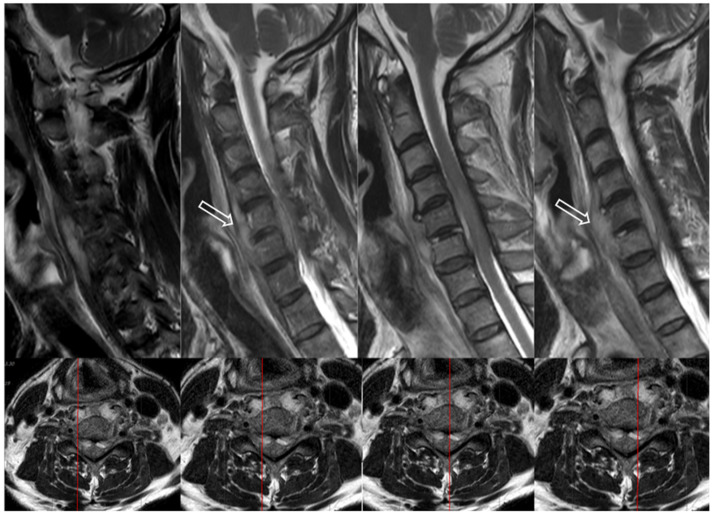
A 64-year-old male patient presented to the emergency room with quadriplegia. Magnetic resonance imaging(MRI) revealed an abscess within both longus coli muscles and discitis at the C5–6 level, along with an epidural abscess extending from C3 to C6, causing spinal cord compression. The white arrows indicate the abscess in the longus coli muscles.
